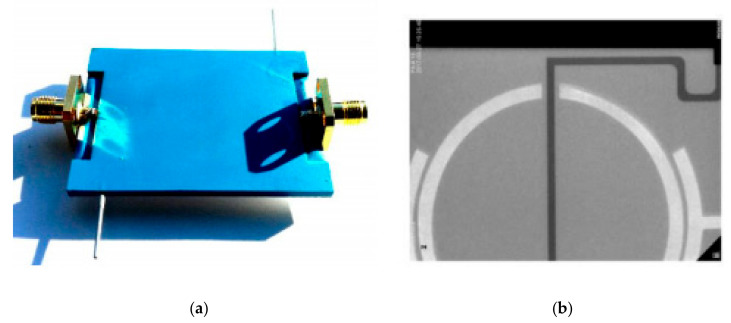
The photo of the manufactured detector (a) and the X-ray image with the microwave circuit of the detector (b) [101].
